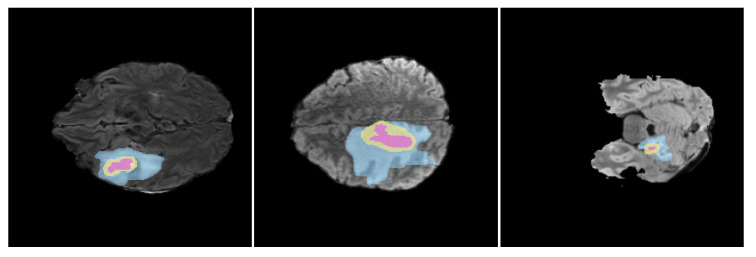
Glioma Structure. This figure displays segmented gliomas on MRI scans, where color coding is used to highlight different tumor regions: blue is the edema (ED), green denotes enhancing tumor (ET), and pink is necrotic cells (NCR). The joint of the ET and NCR areas represents the tumor core region (TC), while the union of the three segmented zones creates the whole tumor (WT).
